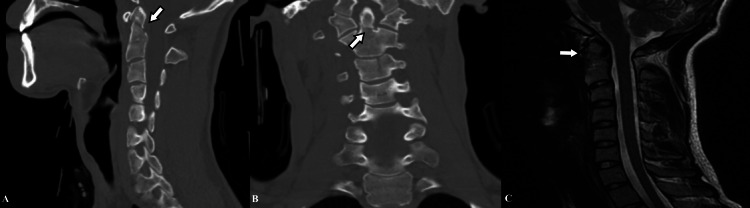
Preoperative cervical CT (A, B) and cervical sagittal MRI (C) images of the patient; fracture line is indicated by a white arrow
